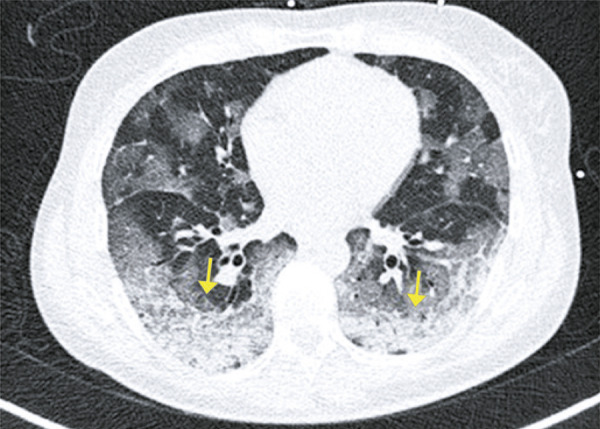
A 51-year-old patient presenting with dry cough and headache for seven days, and fever, for 5 days. Computed tomography showing diffuse ground-glass opacities, associated with peripheral consolidations in the lower pulmonary lobes
